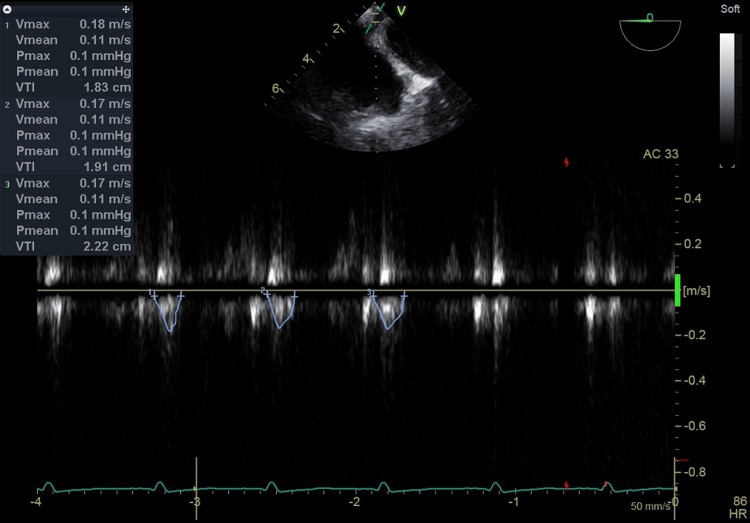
The VTI of CS blood flow measured by the TEE pulse Doppler during heart displacement The VTI value during heart displacement was 4.80 cm (HR 86 beats/min) calculated as the average of three heartbeats excluding premature contractions. In addition, the TEE setting was set to Frames per second 9, Frequency 3.1 MHz, Power 0 dB, Gain -2 dB, Velocity scale 1.5 m/s, Sample volume 4.0 mm, Sample volume depth 0.7 cm and Sweep speed 50 mm/s. VTI: velocity time integral, CS: coronary sinus, TEE: transesophageal echocardiography
